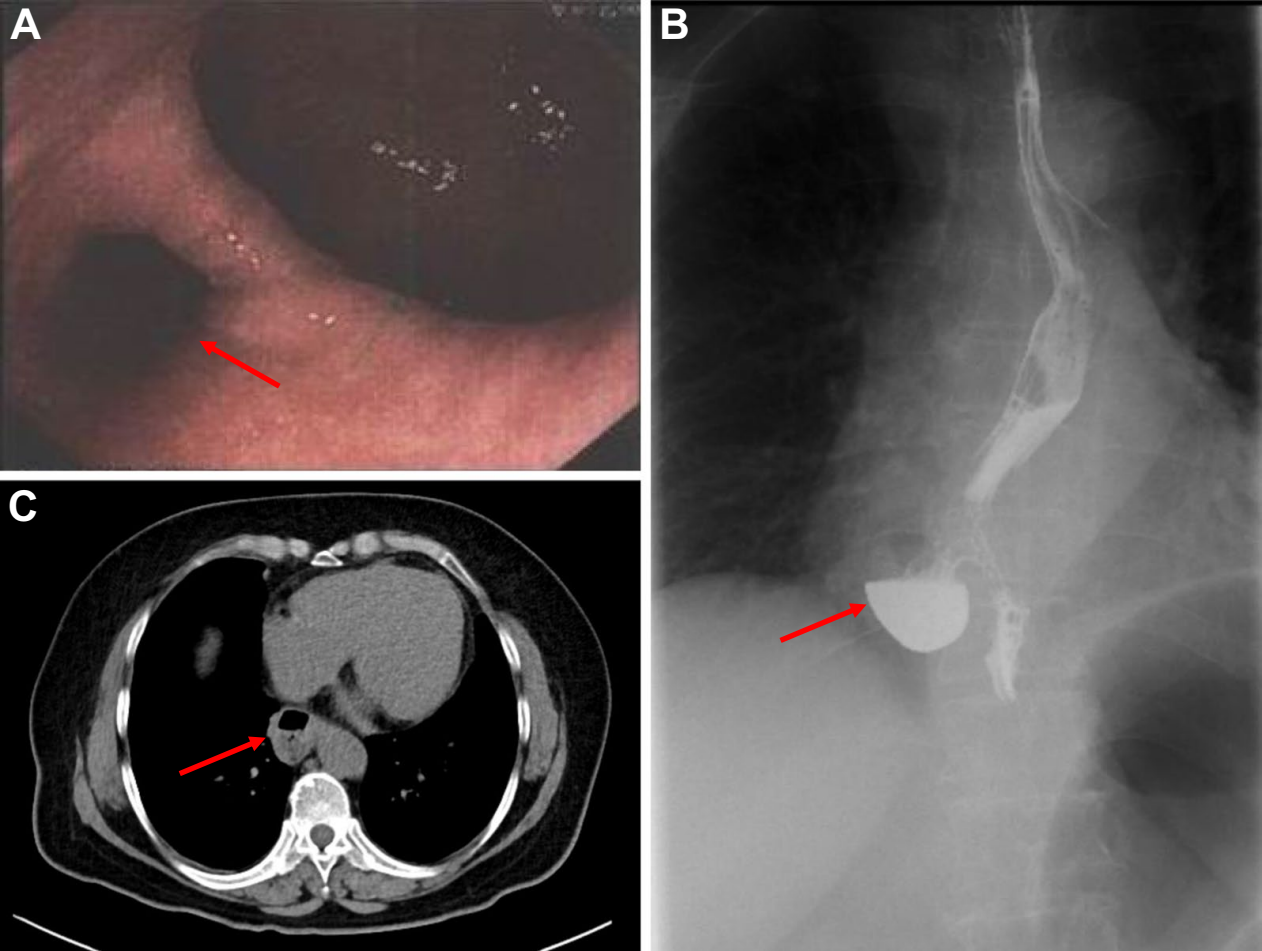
A Upper gastrointestinal endoscopy showed the diverticulum 30 cm from the incisor line. B Upper gastrointestinal contrast examination revealed a diverticulum on the right wall, and barium remained in it. C Chest enhanced computed tomography revealed an epiphrenic diverticulum with a diameter of 35 mm
